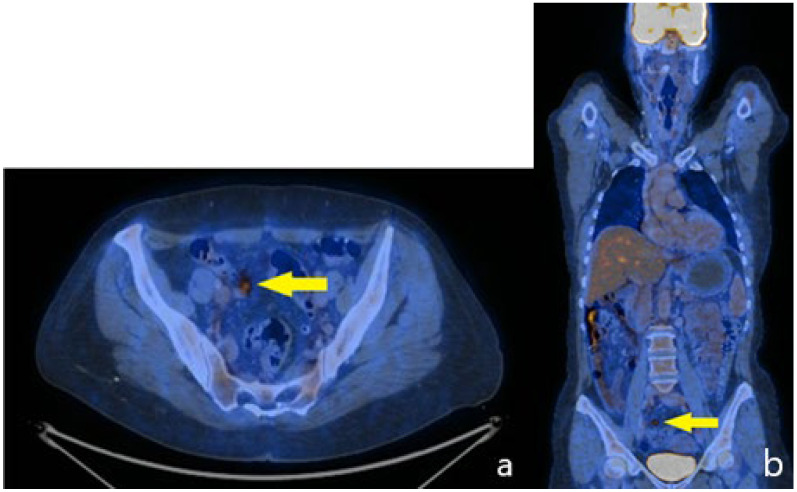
PET CT—postoperative aspect at 6 months, one hot nodule on the right iliac vessels—arrow (a) Transversal view; (b) Coronal view.
